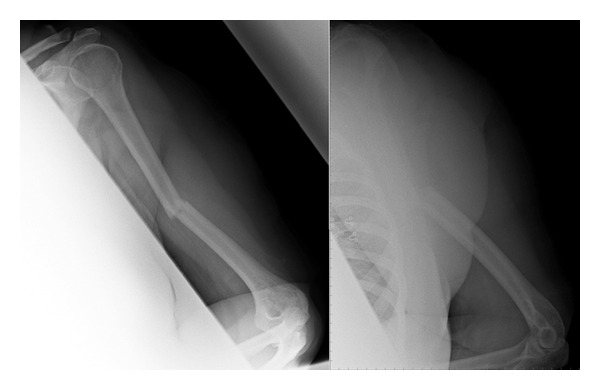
AP and lateral radiographs of midshaft humeral fracture.
